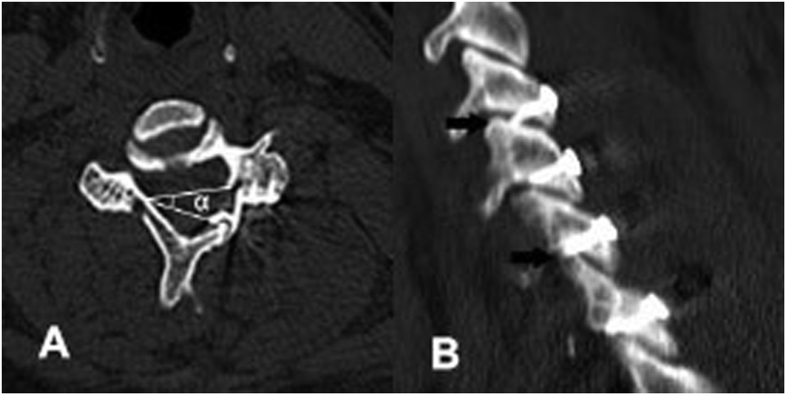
Radiographic measurements. (A) The angle α indicates the laminoplasty opening angle. (B) Facet joints were destroyed by the miniscrews (the black arrows).
